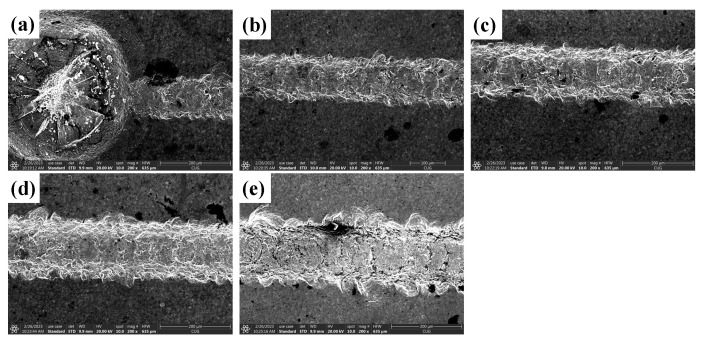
Surface morphology of ultrasound (ultrasound amplitude 8.07 μm) scratches at different positions. (a) show the surface morphology at the start of the scratch; (b–d) show the sur-face morphology at the middle of the scratch; (e) show the sur-face morphology at the end of the scratch.
